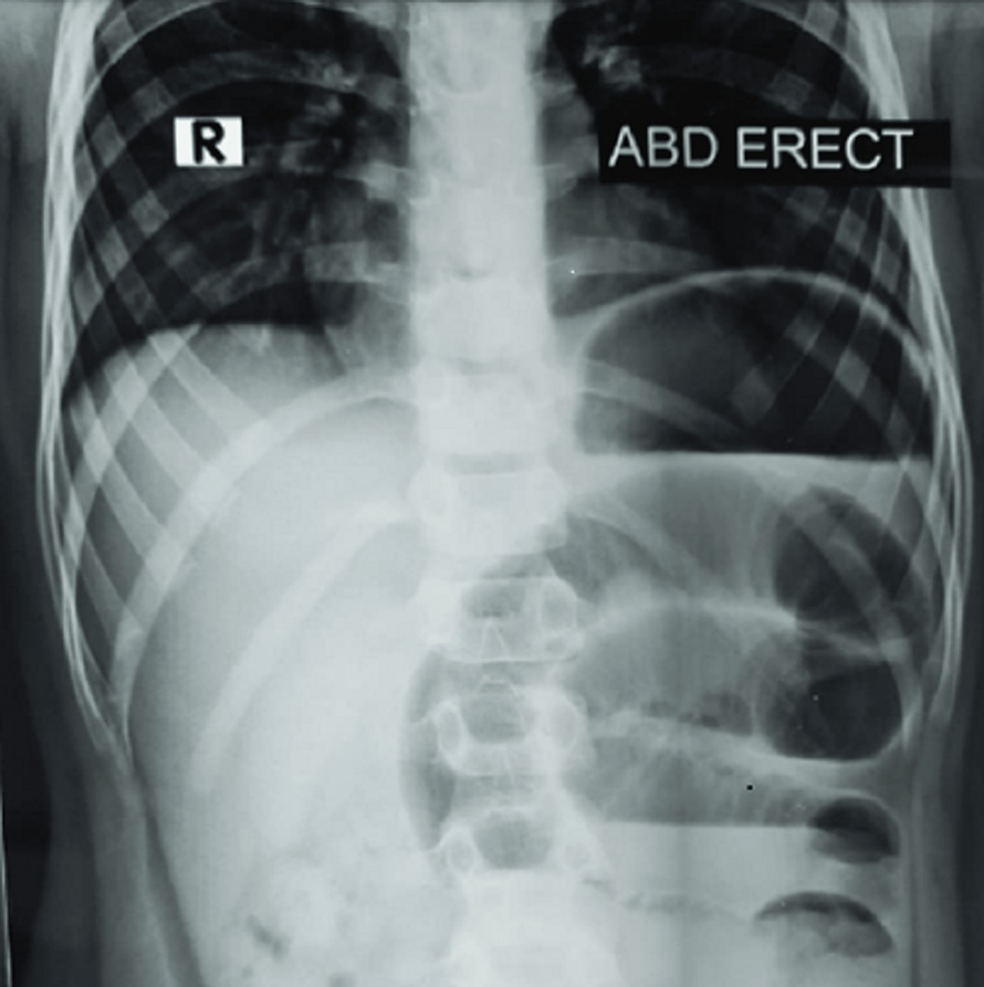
X-ray abdomen with features of intestinal obstruction
